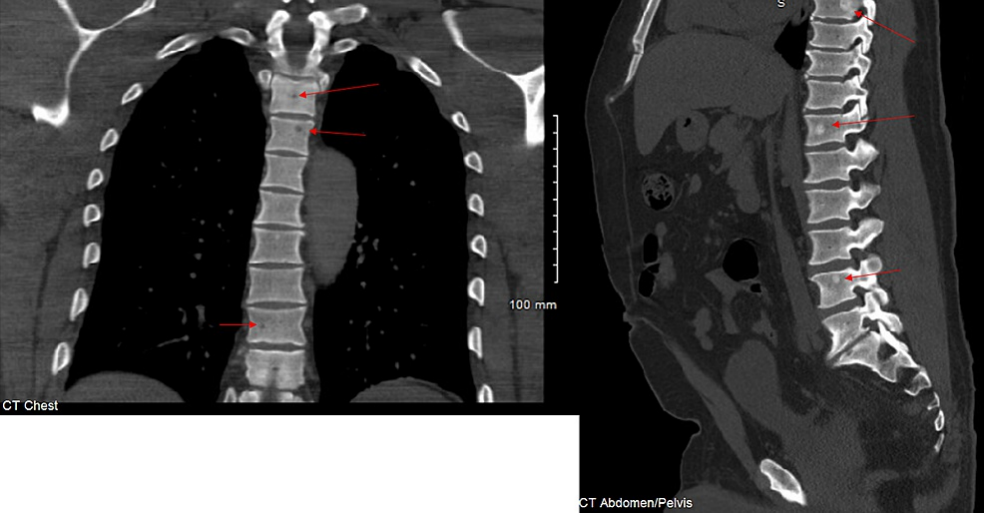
CT scan (bone window): bone lesions likely secondary to metastatic prostate cancer.
